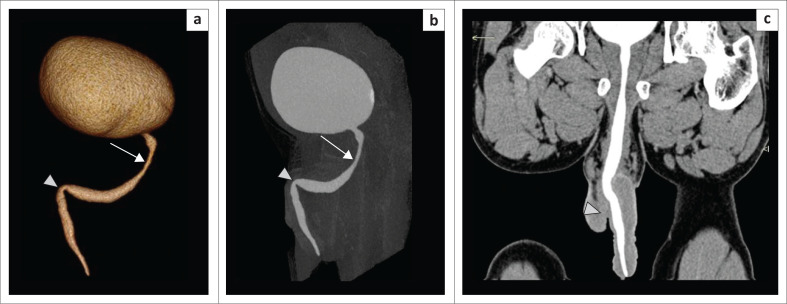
Multidetector computed tomographic urethrography (MDCTU) of a normal urethra: (a) volume rendering technique image in the sagittal view, (b) maximum intensity projection image in the sagittal view and (c) curved multiplanar reformatted (MPR) image. The urethra is seen arising from the bladder neck as a contrast-filled tubular structure and opening into the external urethral meatus. The membranous urethra is the narrowest part with near-parallel walls, at the level of the external urethral sphincter. The bulbar urethra is the widest portion having a normal coned appearance at the bulbomembranous junction (arrow). The bend or kink at the peno-scrotal junction (arrowhead) is normal since the penis is not stretched on MDCTU. The curved MPR image (c) confirms the kink as normal calibre.
